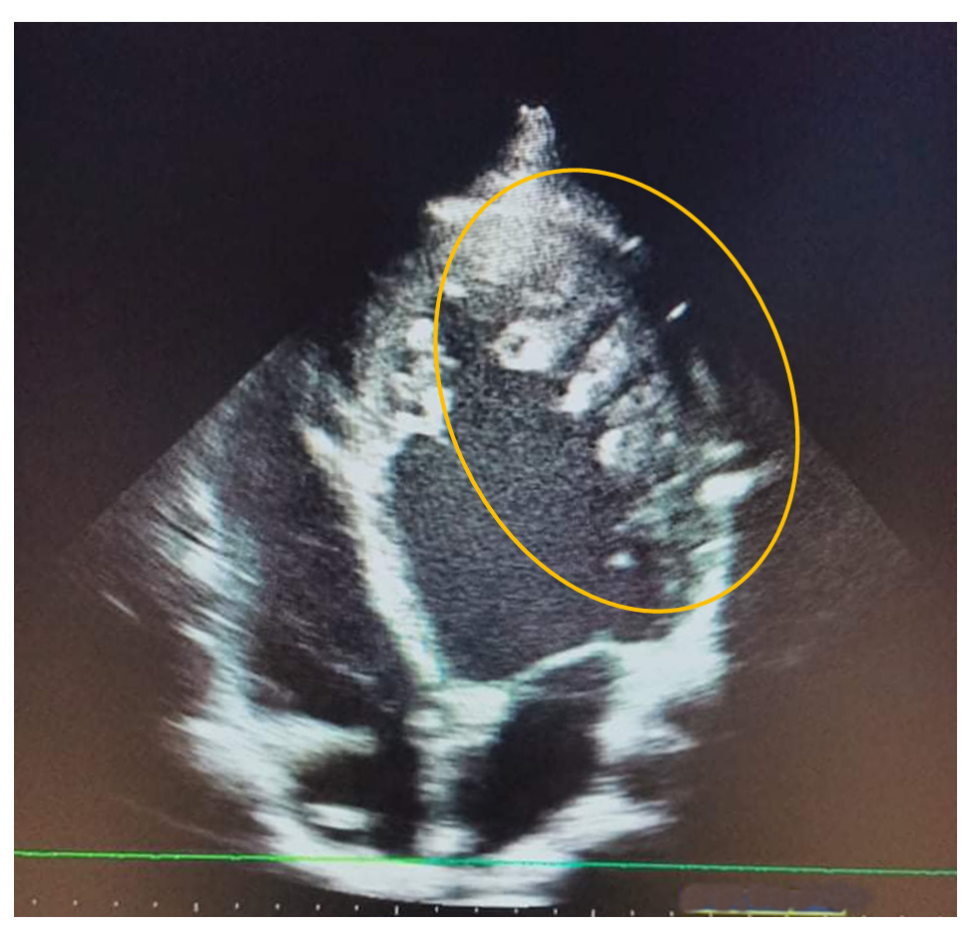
Transthoracic echocardiography aspect of the left free wall showing non compacted trabeculation (circle).
